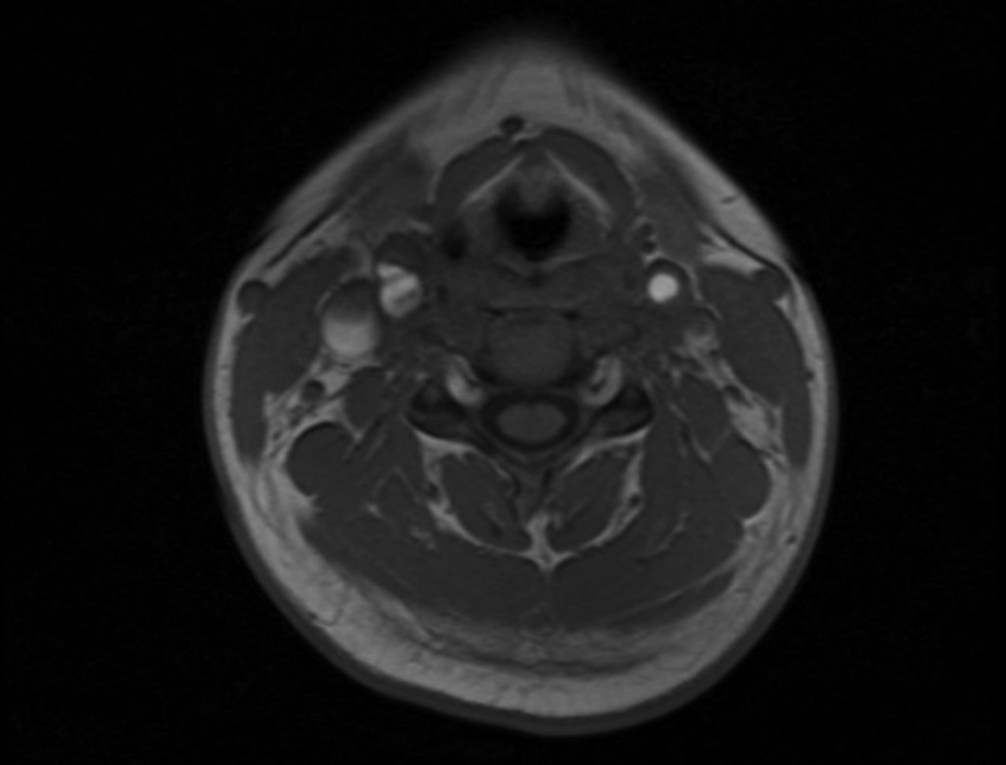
T*_1_ weighed MR image of the neck showing hypointense perivascular thickening around right carotid bulb.
